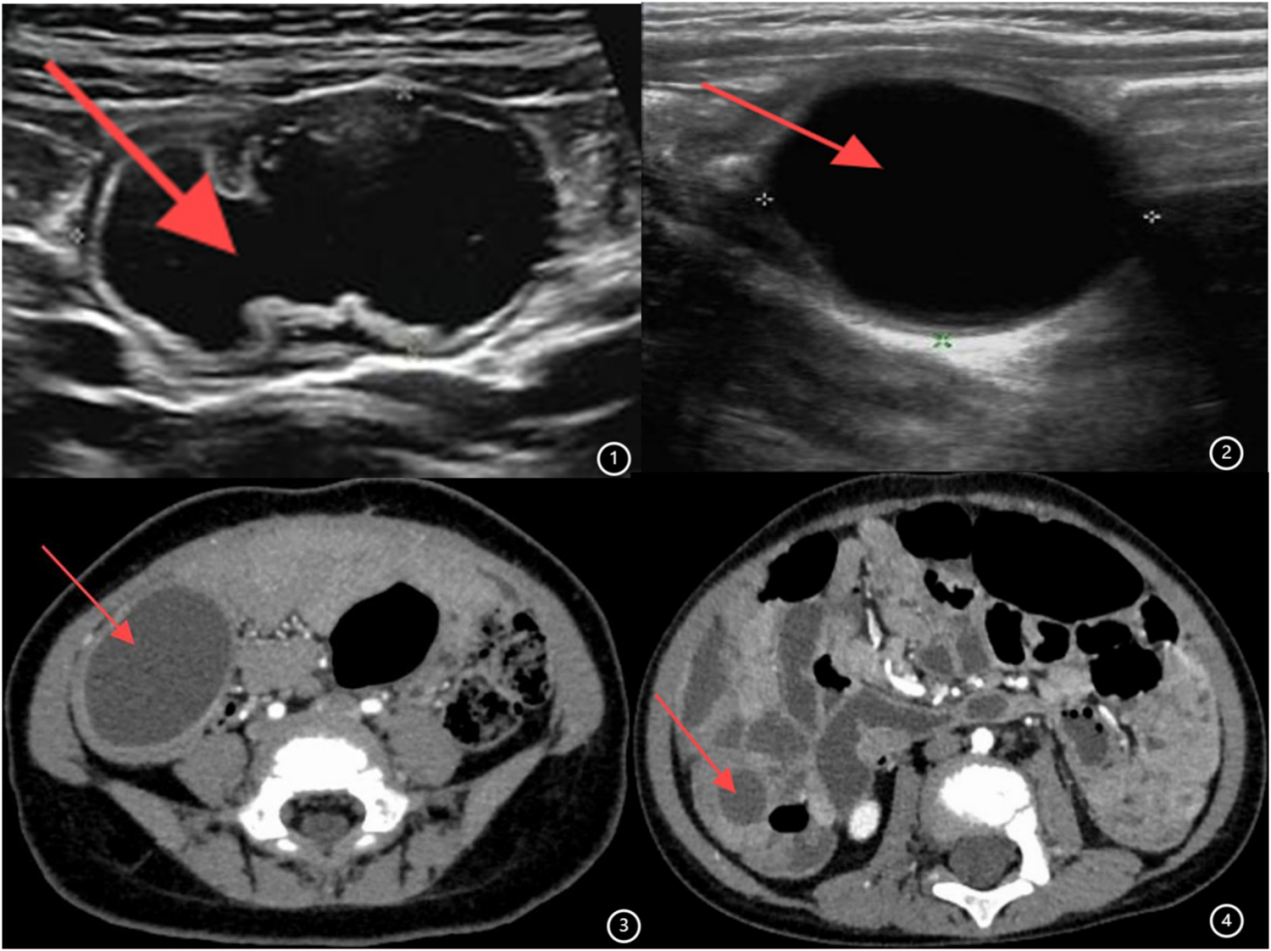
(1,2) Ultrasound image of intestinal duplication malformation. (3,4) Enhanced CT image of intestinal duplication malformation.
